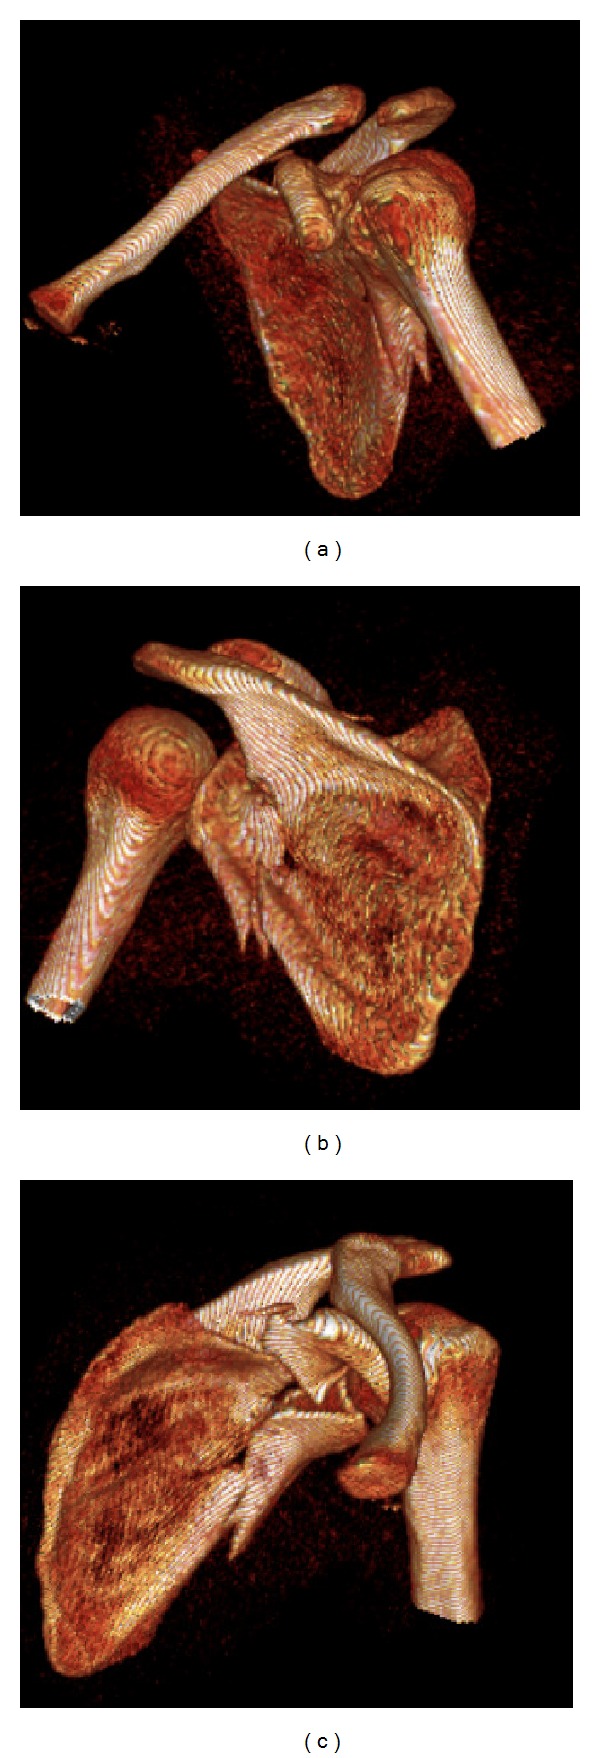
Three-dimensional reconstructions of the left shoulder CT scan.
